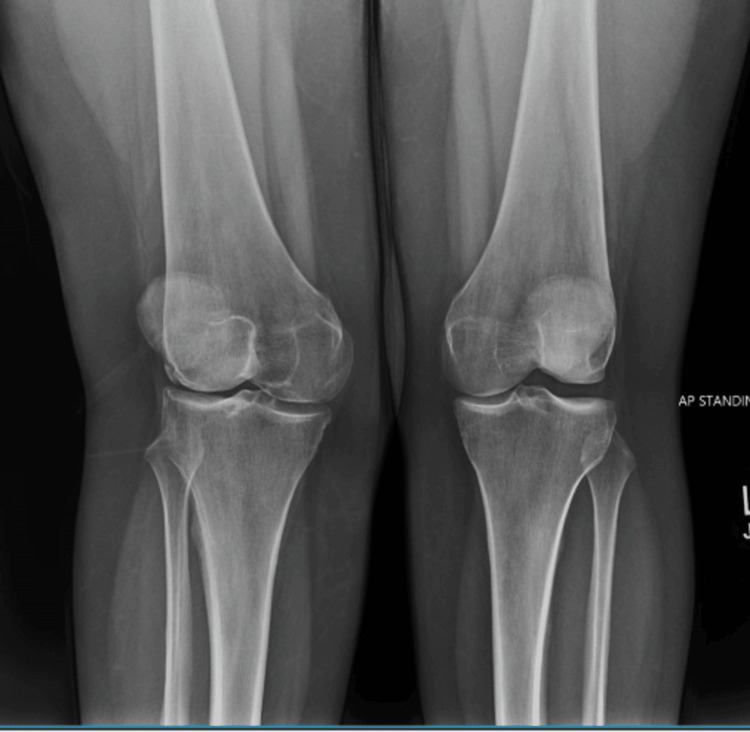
Standing anteroposterior radiograph of both knees demonstrating asymmetric joint space narrowing
